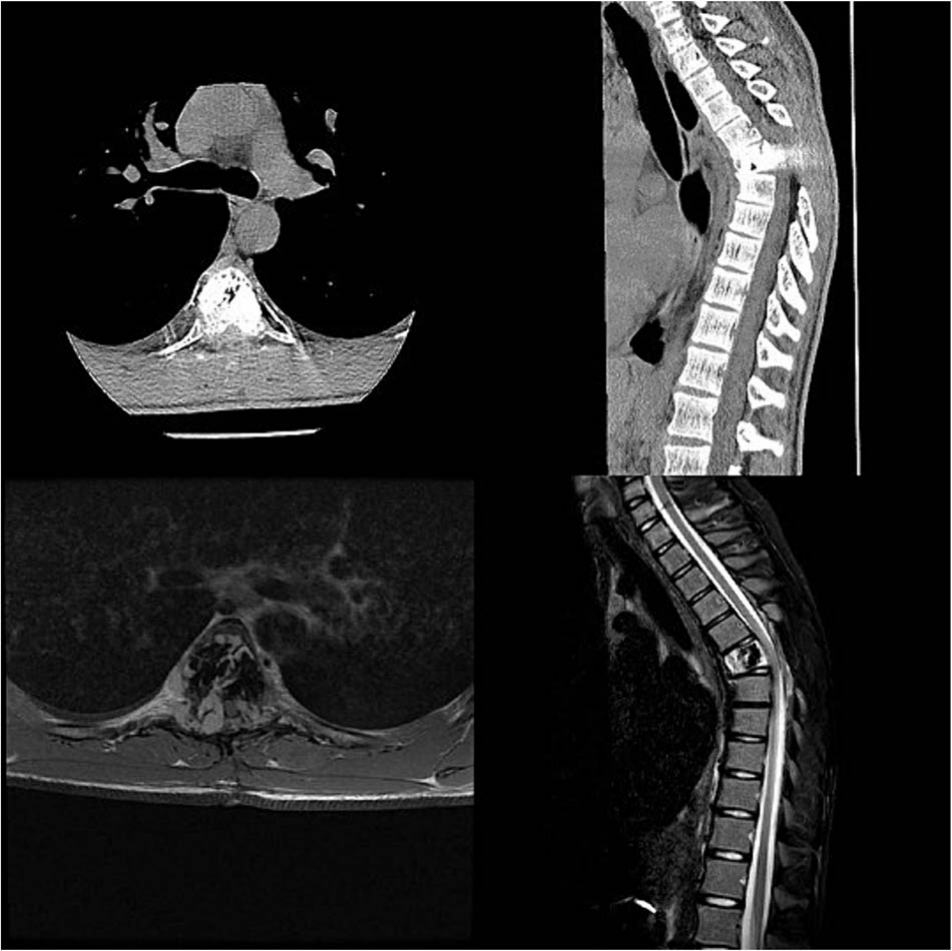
Follow-up CT imaging revealed recurrent AVH of the Th6 vertebrae, MRI scan showing AVH expanding into the spinal canal and causing myelopathy.
